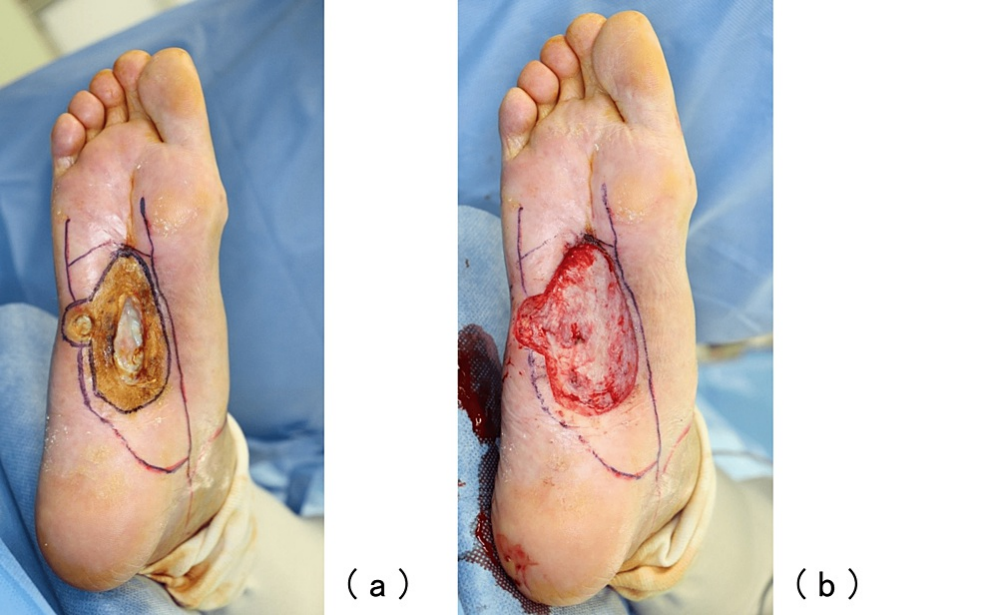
The first stage of operation The plantar artery was marked on the skin using ultrasonography before the operation, and considerable attention was paid not to injure the artery during the operation (a); at the end of the first stage of operation (b).
